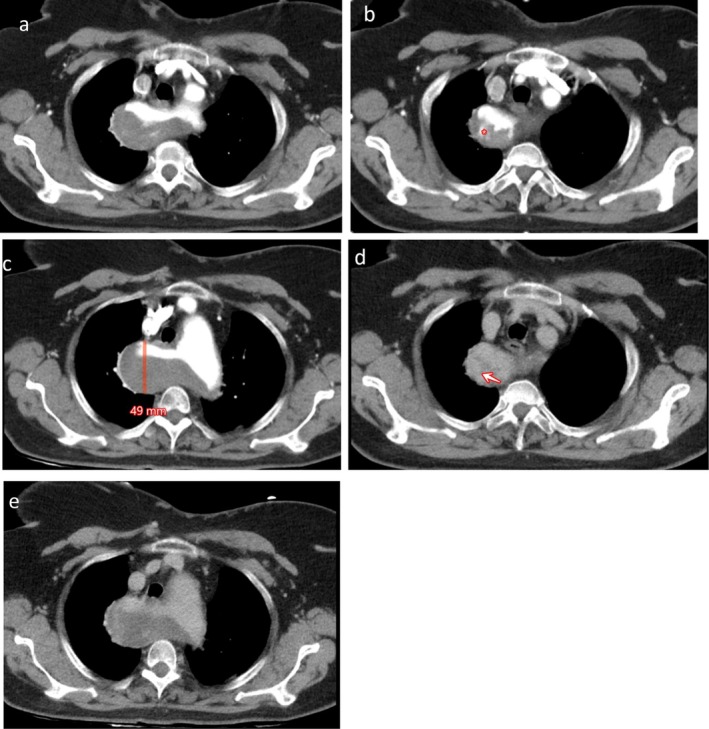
The 5‐week follow‐up CT scan of the same patient. (a–c) The arterial phase (CTA) axial images under soft tissue/mediastinal window settings, reveal the Kommerell aneurysm with a maximum diameter of 49 mm, irregularity of the inner wall of ARSA, and a hyperdense crescent sign in the mural thrombus (*). (d, e) The delayed phase images (7 min), show increased attenuation of the mural thrombus (arrow).
